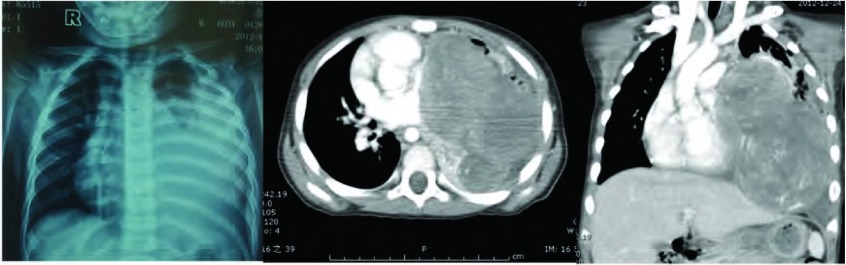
胸片及胸部CT增强示左侧胸腔有一大小约10 cm×10 cm×8 cm哑铃状分叶肿物，增强检查病灶可见不规则强化影。 Chest roenrtgenography and contrast enhanced CT scan of the chest: a giant dumb-bell sublobe mass (10 cm×10 cm×8 cm) in the left thoracic cavity. The solid portion of the mass shows a slight contrast enhancement.
